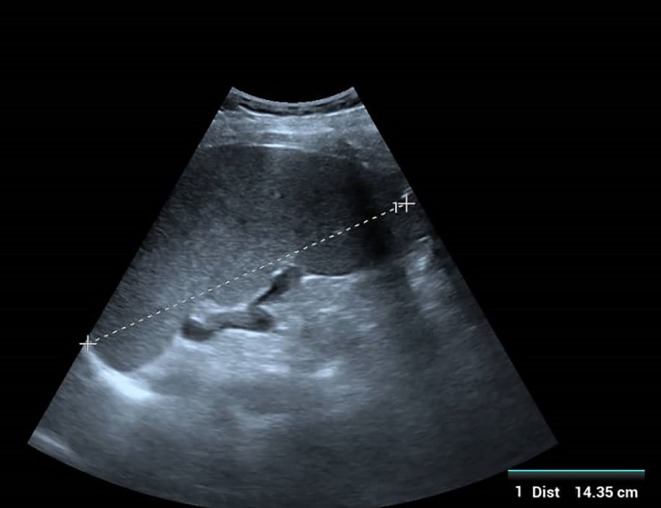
Abdominal ultrasound demonstrating marked splenomegaly (spleen length 14.35 cm) with homogeneous echotexture. No focal mass identified.
